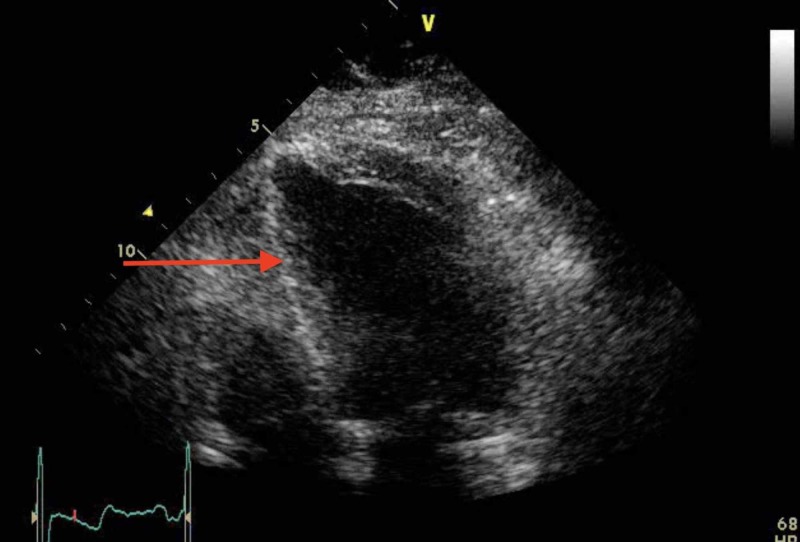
Echocardiogram on admission. Image taken during ventricular contraction. The red arrow highlights akinesia of the inferior segment with a severely akinetic apex.
